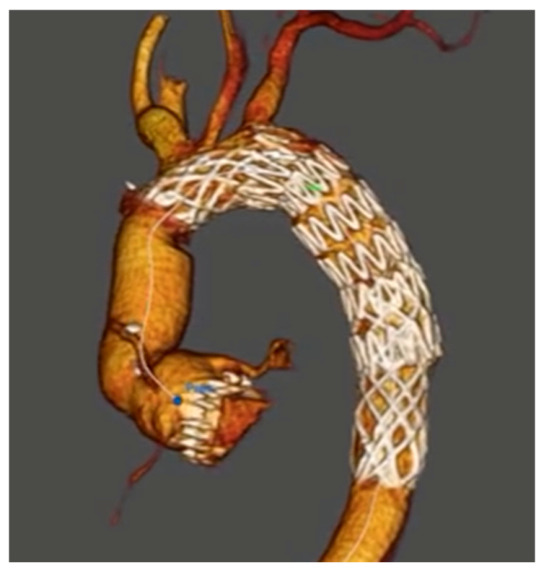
Post-operative computer tomography (CT) aortogram. Figure legend: Post-operative computed tomography aortogram showing well-seated AMDS and restored true lumen flow.
